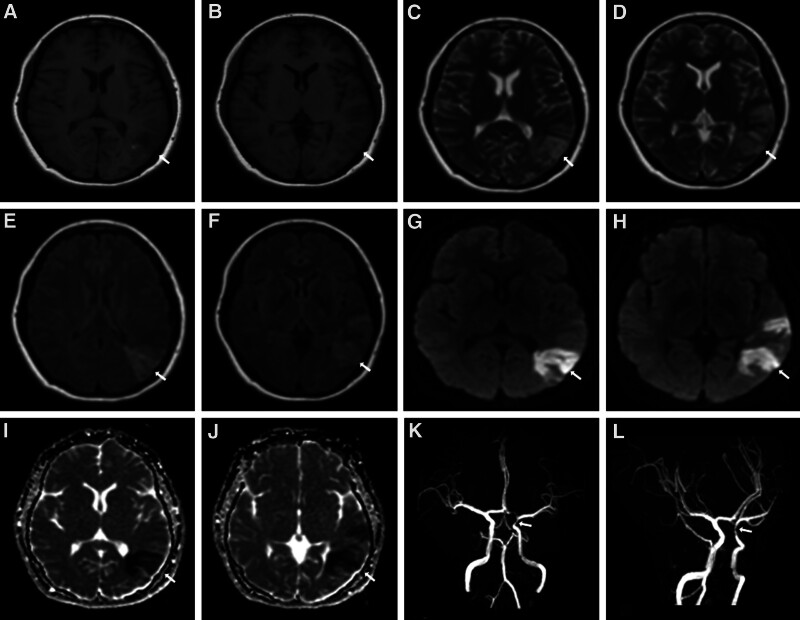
Brain MRI at admission. Axial T1-weighted sequence (A, B), T2-weighted sequence (C, D), FLAIR sequence (E, F), DWI (G, H), and ADC (I, J) sequences were imaged and showed abnormal hyperintensities signals in the left head of temporal, parietal, and occipital lobes. The partial stenosis of the left internal carotid artery in the cerebral MRA (K, L). ADC = apparent diffusion coefficient, DWI = diffusion-weighted imaging, FLAIR = fluid attenuated inversion recovery, MRA = magnetic resonance angiography, MRI = magnetic resonance imaging.
